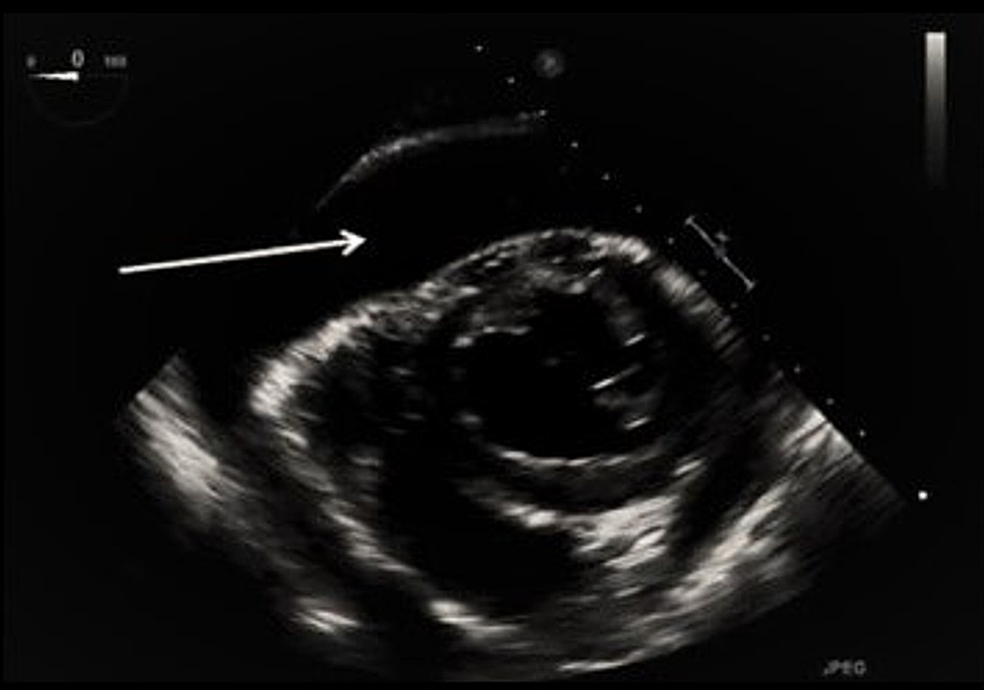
Bedside transesophageal echocardiogram (TEE) with pericardial effusion A transgastric short-axis view demonstrated right atrial and right ventricular collapse during the majority of the cardiac cycle with a significant reduction of venous flow.
